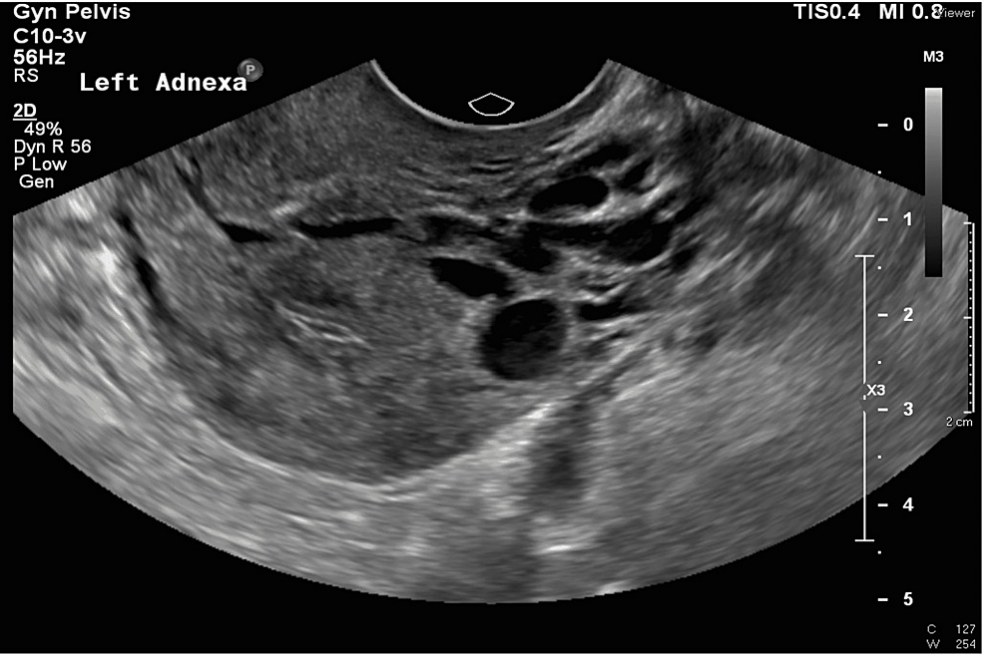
Pelvic ultrasound greyscale demonstrates dilated left adnexal veins
